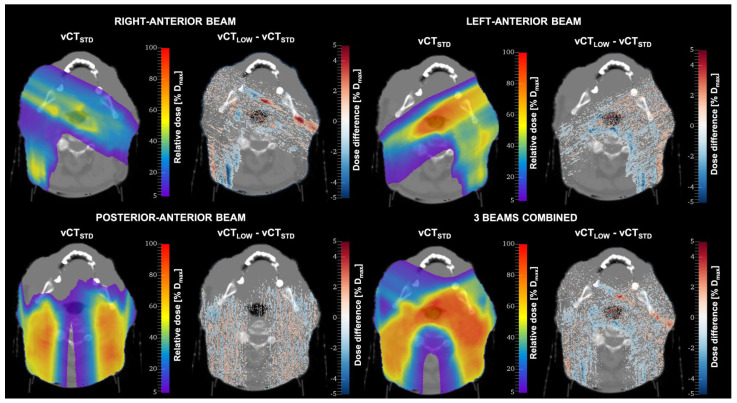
Dose distributions calculated for one patient in standard-protocol CT images (vCT_STD_) and the difference between the dose distributions calculated in vCT_STD_ and low-dose CT (vCT_LOW_).
